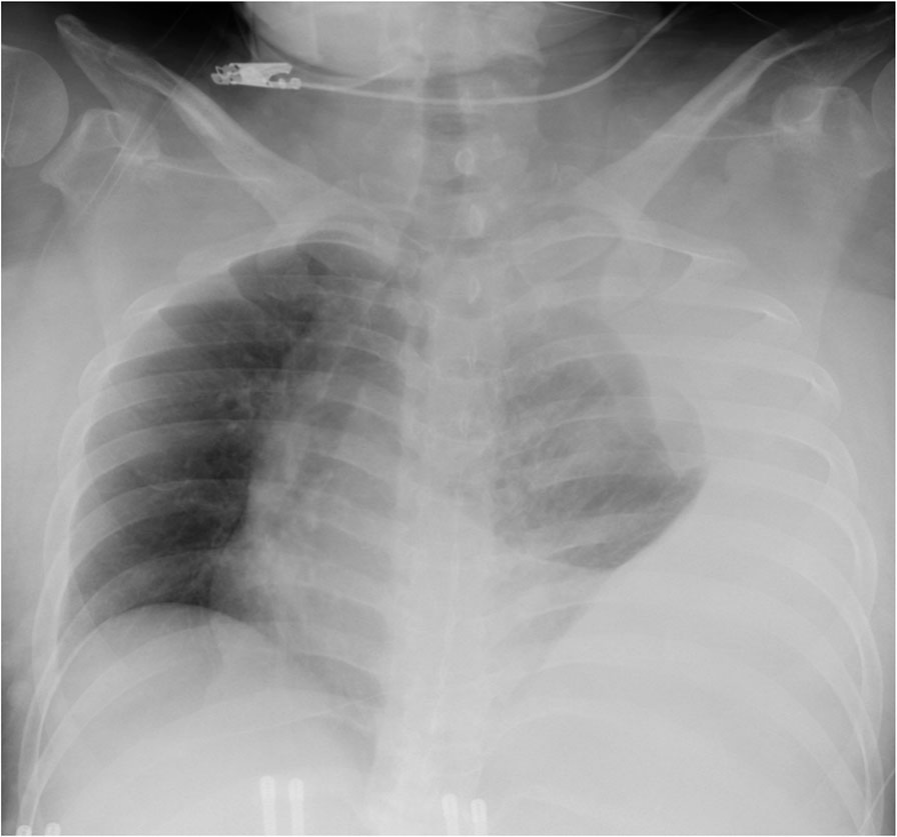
Frontal chest radiograph showing massive left pleural fluid collection with a mediastinal shift to the right
